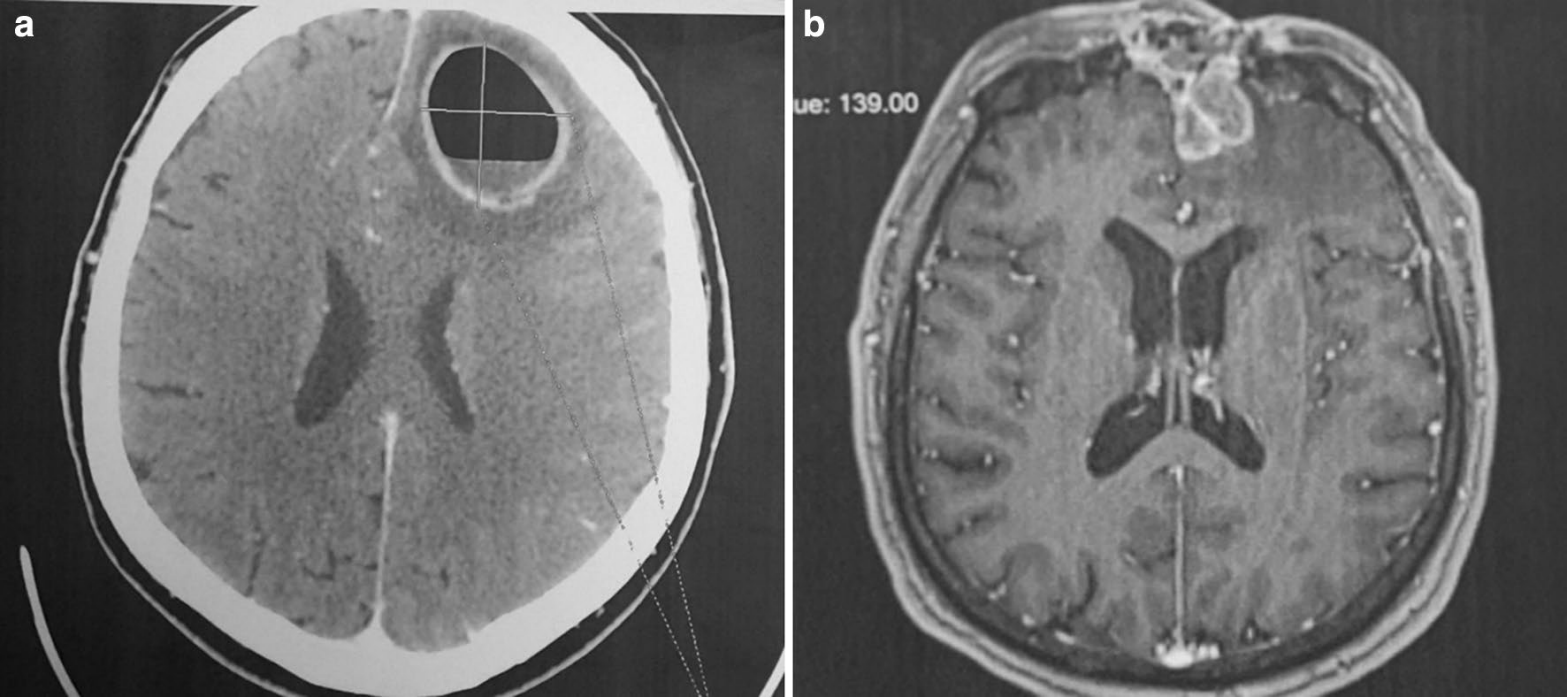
a MRI of the cranium in a 58-year-old man. The abscess with a distinct size of 38 × 28 mm in the left frontal lobe produces a marked displacement and compression of the left chamber. b MRI of the cranium in the same patient hospitalised after 12 months due to the recurrence of symptoms. Irregular multichamber abscess in the left frontal lobe measuring 32 × 24 × 16 mm
